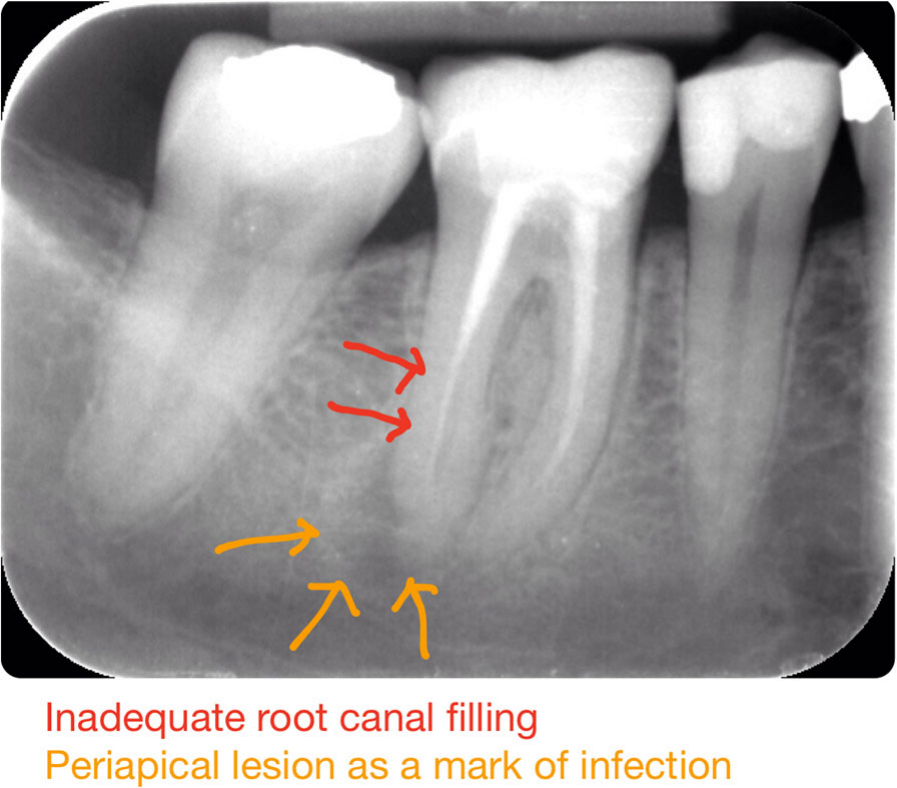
Example of student’s annotated dental X-ray
